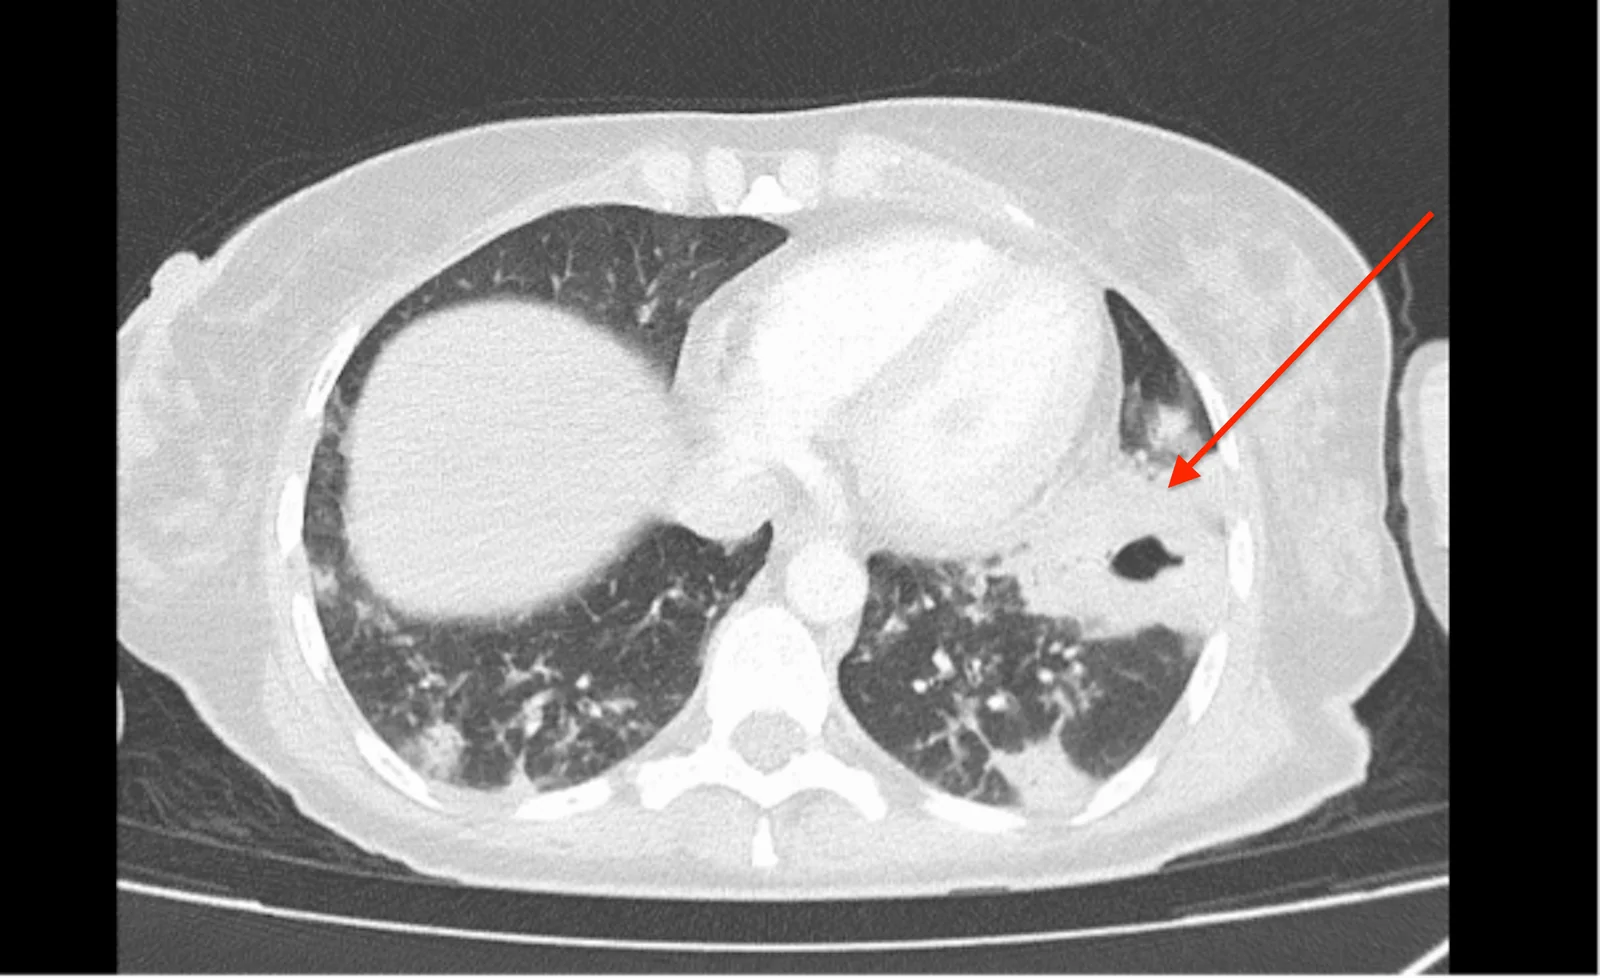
Contrast-enhanced computed tomography (CT) thorax demonstrating cavitating lung abscess Axial CT image demonstrating a thick-walled cavitating lesion containing an air-fluid level within the left lower lobe (arrow), consistent with a lung abscess secondary to septic embolisation. Surrounding consolidation and bilateral bronchopneumonic changes were also evident.
